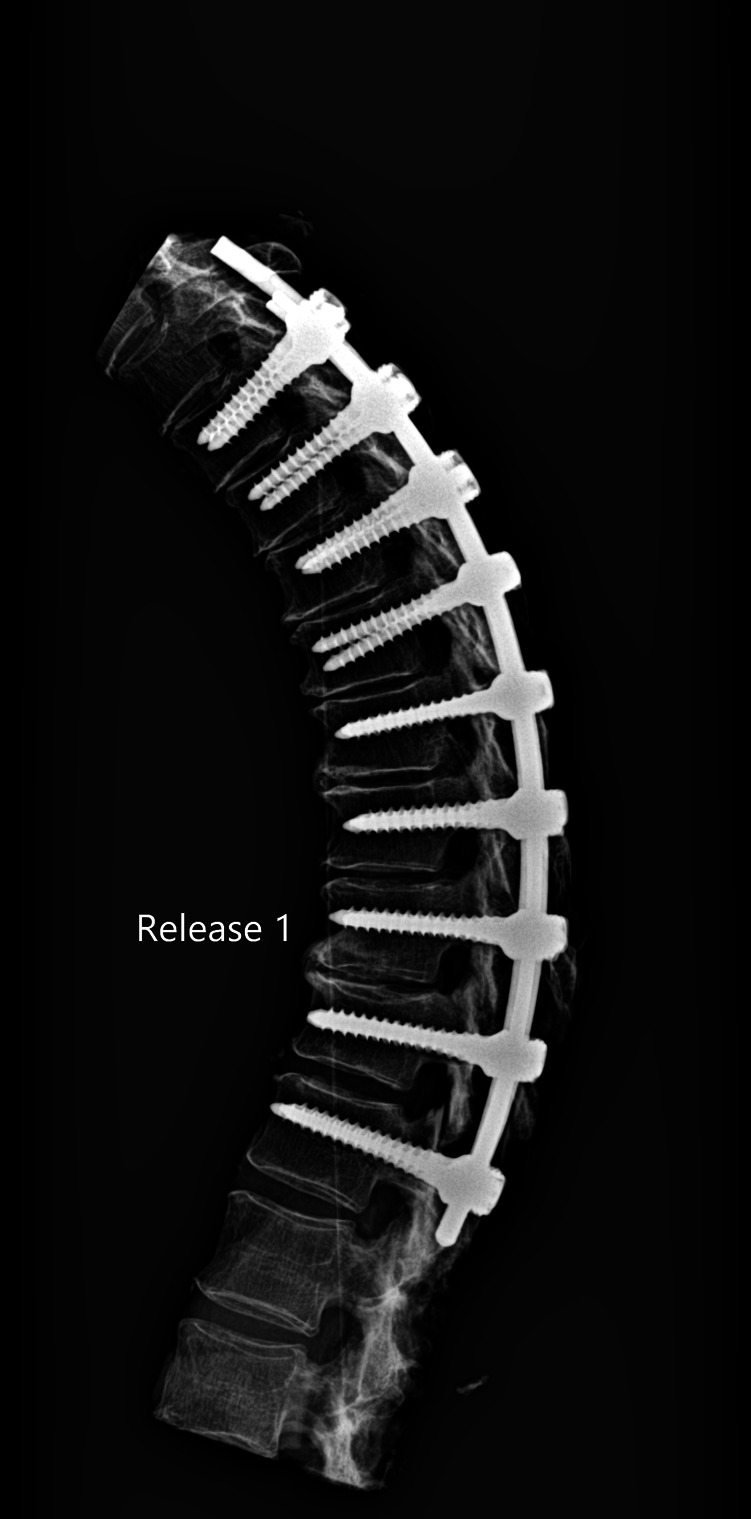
Lateral radiograph of sequential release of the thoracic spine with pre-contoured rods to induce thoracic kyphosis: Release 1—ISL transected.
